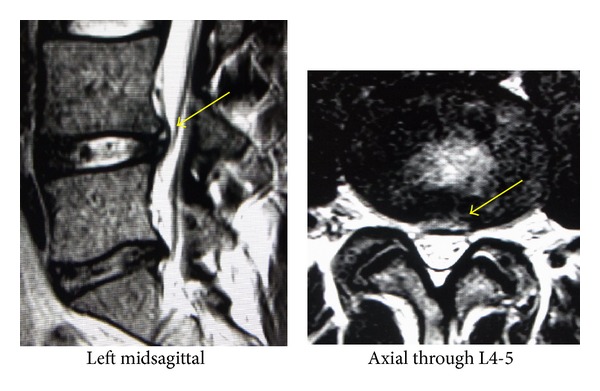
T2-weighted MRI findings on the second visit. Sagittal and axial images both show that the size of the disc protrusion is similar to that in the first MRI. This time, however, the HIZ is obvious.
